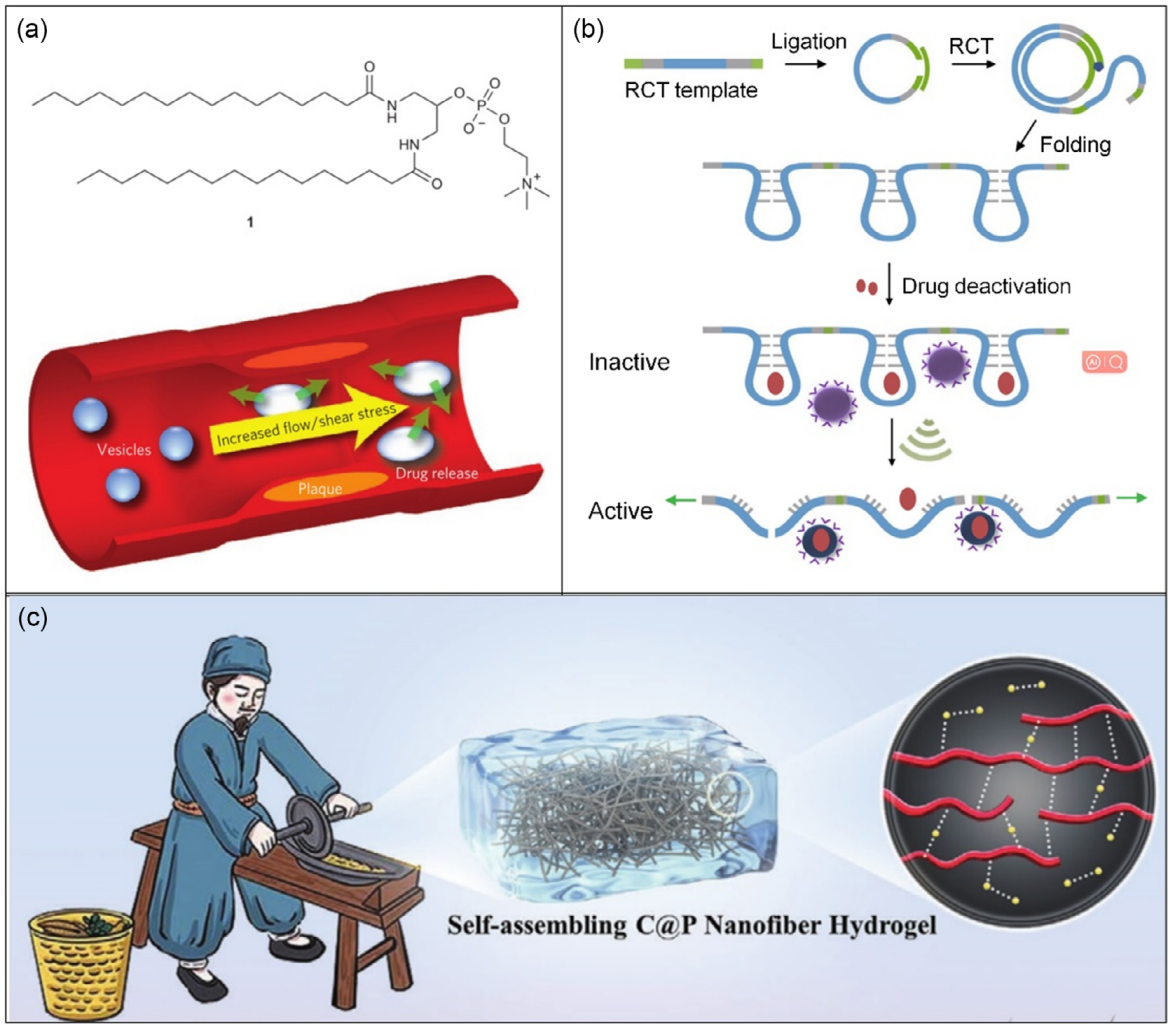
Mechanical activation of therapeutics. a) Molecular structure of 1,3‐diaminophospholipid and self‐assembled liposomes that undergo shape deformation in shear flow. b) Polyaptamers designed from a circular template are loaded with neomycin B, which are released by ultrasound‐induced stretching and bond scission. c) Mechanical grinding promotes the molecular collision and accelerate self‐assembly into therapeutic hydrogels. a) Adapted with permission.^[^
